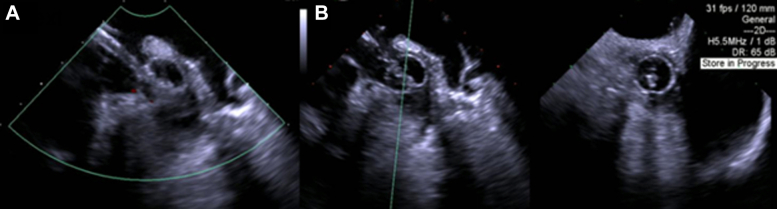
Transesophageal Echocardiogram Confirmation of SafeCross LAA Os Occlusion SafeCross balloon inflated further until complete occlusion of left atrial appendage (LAA) preventing thrombus embolization, in multiple views (A and B).
